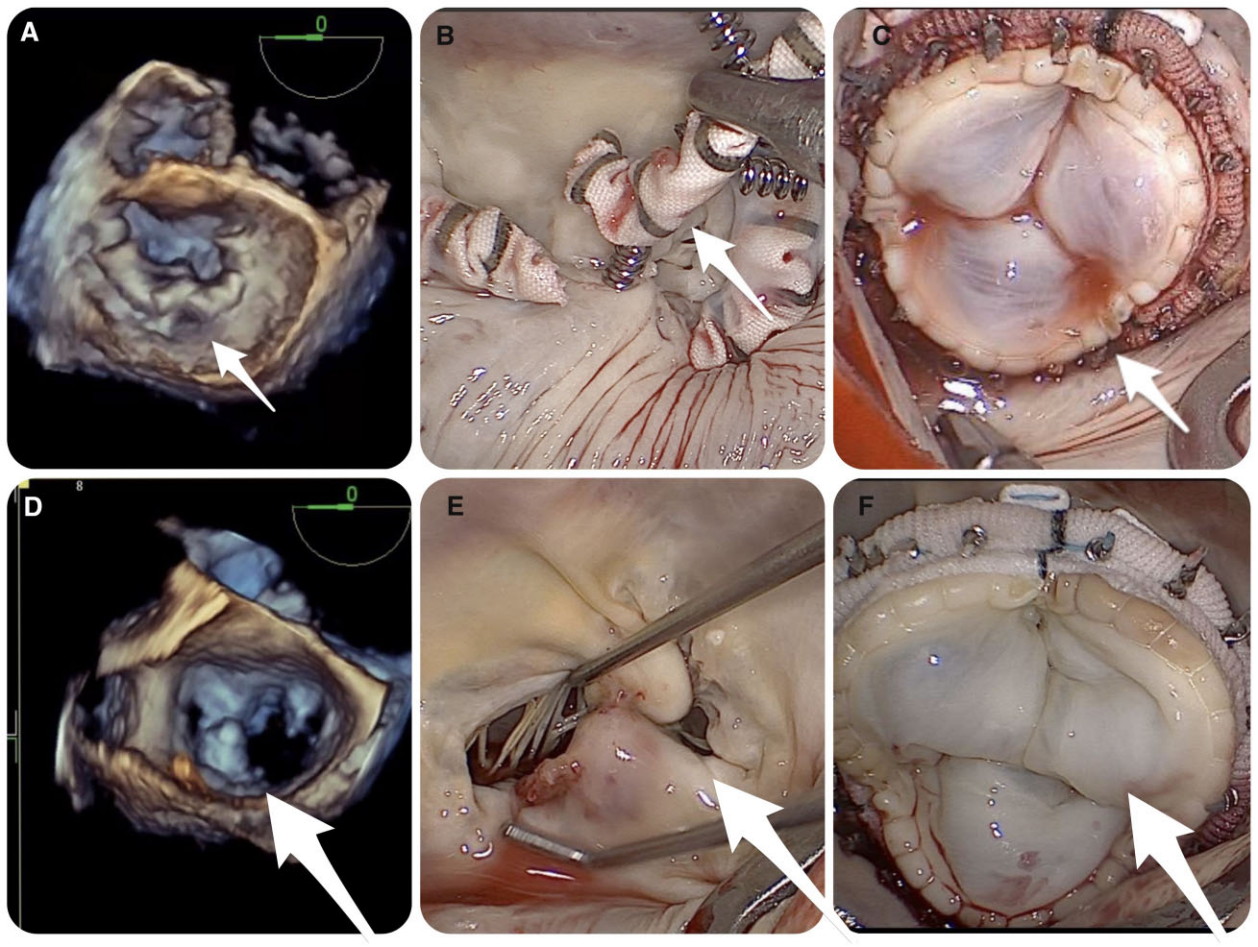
(A) Explantation of a Cardioband device: Perioperative transoesophageal echocardiography showing partial detachment of the device; (B, C) explantation with the cut-and-unscrew technique and valve replacement. (D) Perioperative transoesophageal echocardiography showing a MitraClip in place and annular dilatation; (E, F) mitral valve replacement with a biological prosthesis following removal of the MitraClip.
